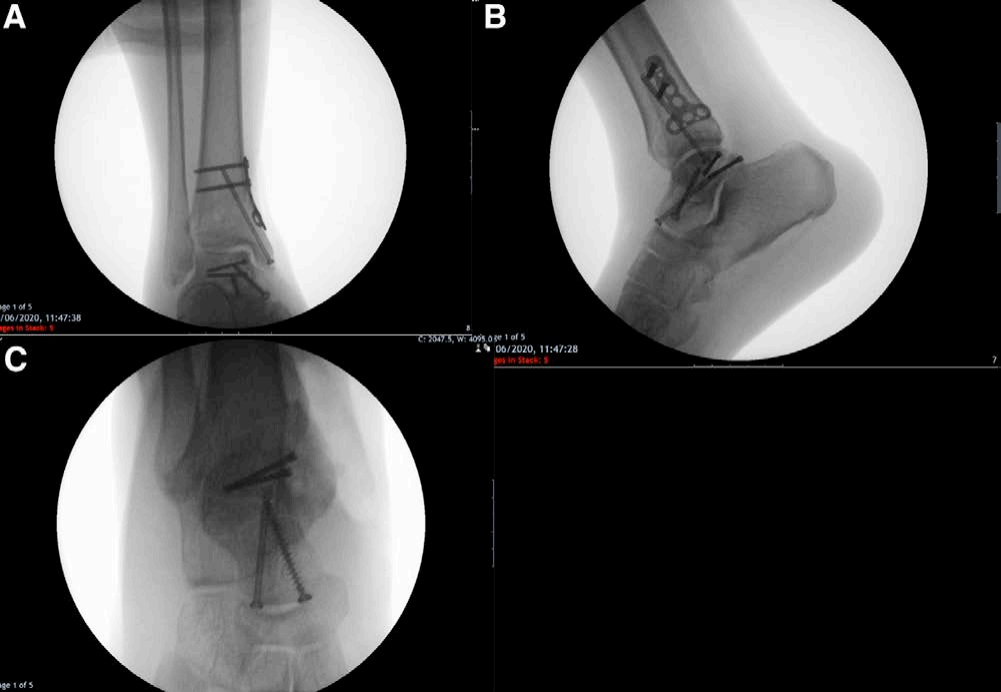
Intraoperative ankle X-rays. (A) AP, (B) LAT, and (C) Canale views confirming satisfactory open reduction and internal fixation of the talus. AP = anteroposterior, LAT = lateral.
